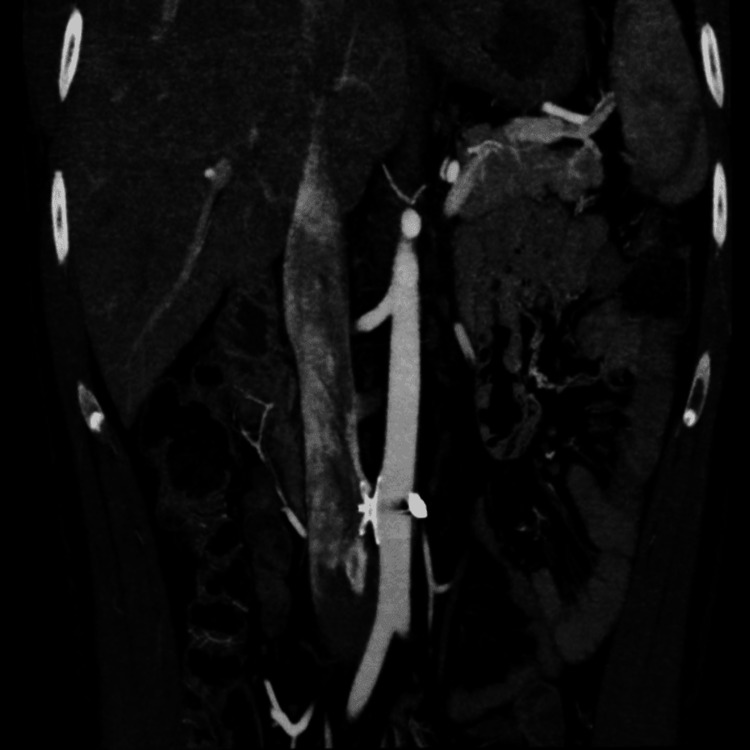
Computed tomography angiography of the abdomen (coronal view) Exclusion of the fistula with mild endoleak and well positioned ASO.
